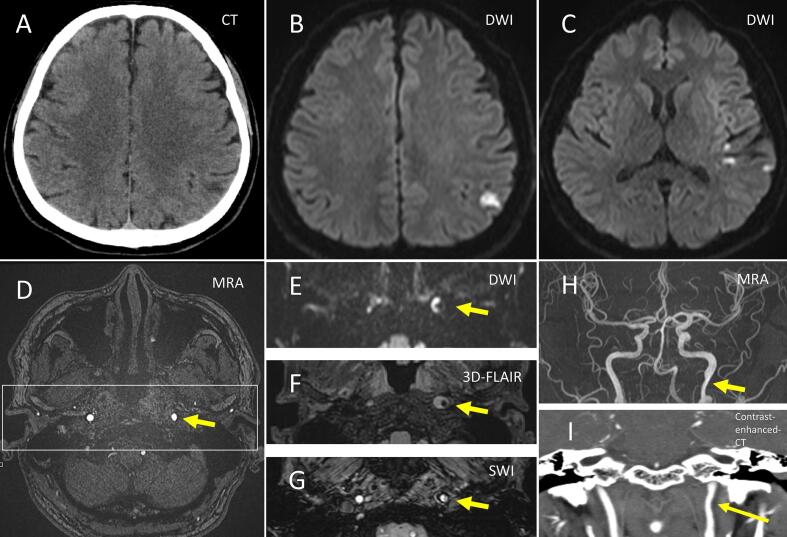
Magnetic resonance (MR) imaging (MRI) of internal carotid artery (ICA) dissection. Computed tomography (CT) reveals a low-density area in the left parietal lobe (A). This low-density area on CT is hyperintense on MRI diffusion-weighted imaging (DWI), indicating an acute infarction (B). Small hyperintensities are observed in the left hemisphere (C). The MR angiography (MRA) source image reveals stenosis of the left ICA (yellow arrow, D). In the area of stenosis, the intramural hematoma is detected as hyperintense on DWI (yellow arrow, E) and 3D-fluid attenuated inversion recovery (yellow arrow, F) and hypointense on susceptibility-weighted imaging (yellow arrow, G). These findings are compatible with those of ICA dissection. Stenosis due to dissection observed on MRA (yellow arrow, H) occurs approximately 2 cm proximal to the base of the skull (yellow arrow, I). (For interpretation of the references to colour in this figure legend, the reader is referred to the web version of this article.)
